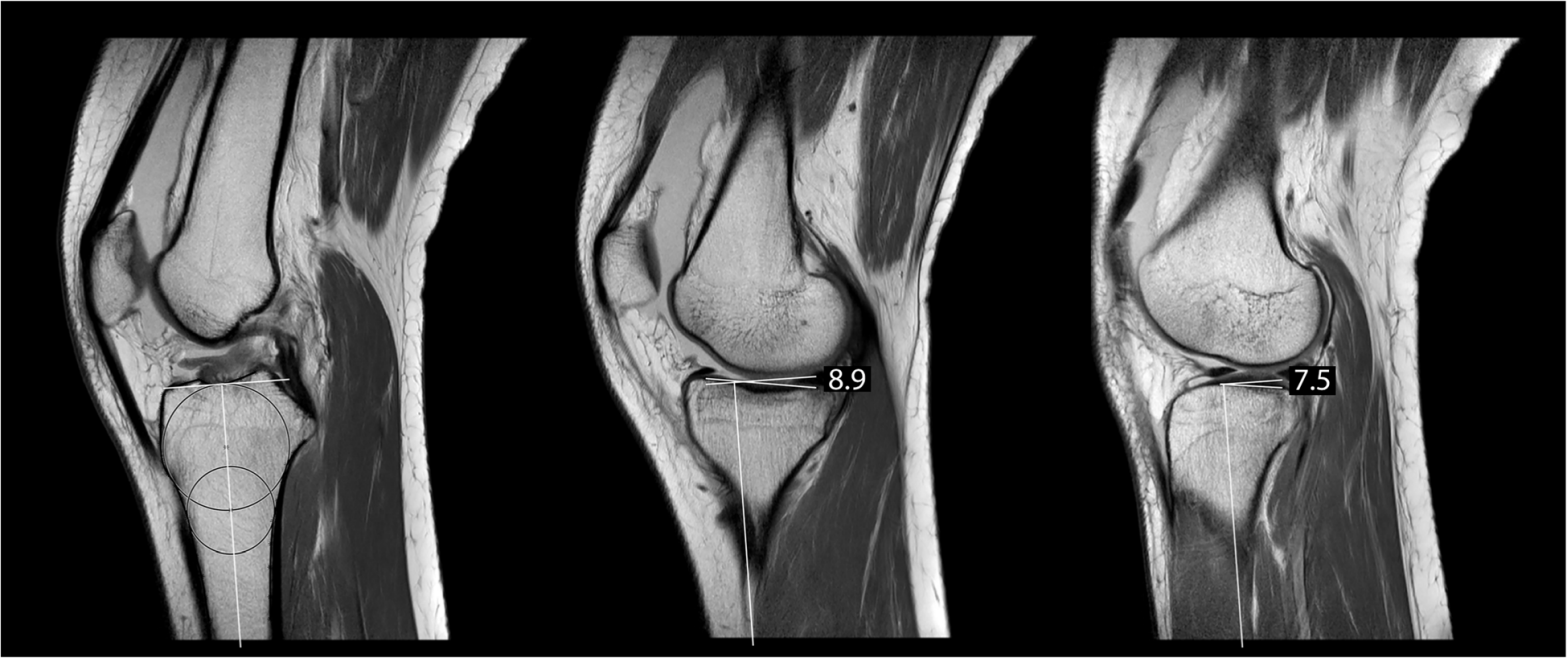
Determination of the medial and lateral PTPA using MRI and the circle method [12, 20]. First, the central sagittal MRI image was found (left image). This image was determined using the following criteria: the anterior and posterior proximal tibia cortices were visible in concave shape and the intercondylar eminence and the posterior cruciate ligament attachment were visible in the image. In this image, a circle was fitted to the proximal tibia, tangential to the cortices. A second circle was fitted distally in the tibia with its centre placed on the first circle. The longitudinal axis was determined by the line connecting the centres of the two circles. Then, the mid-sagittal images of the medial and lateral femoral condyles were selected (middle and right image). The angle between the line connecting the anterior and posterior articular surface of the posterior tibia plateau and the line at right angles to the longitudinal axis of the tibia on both medial and lateral images were the MPTPA and LPTPA respectively
